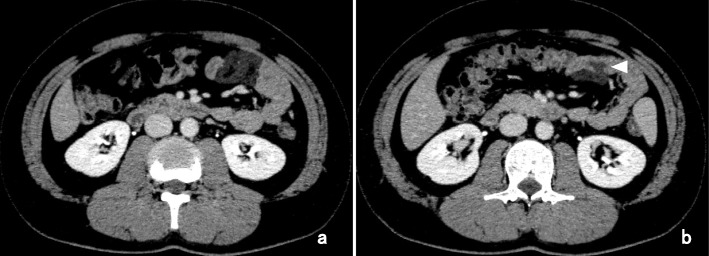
Findings of the CT scan performed in March. a The low-density mass showed no increase in size, and the mass did not show contrast in the enhanced phase. Some lower-density components were observed inside of the low-density mass. b CT enhancement revealed apparent tumor invasion of the wall of the jejunum (white arrowhead) without ischemia in the adjacent jejunum or dilatation of the oral side of the bowel
